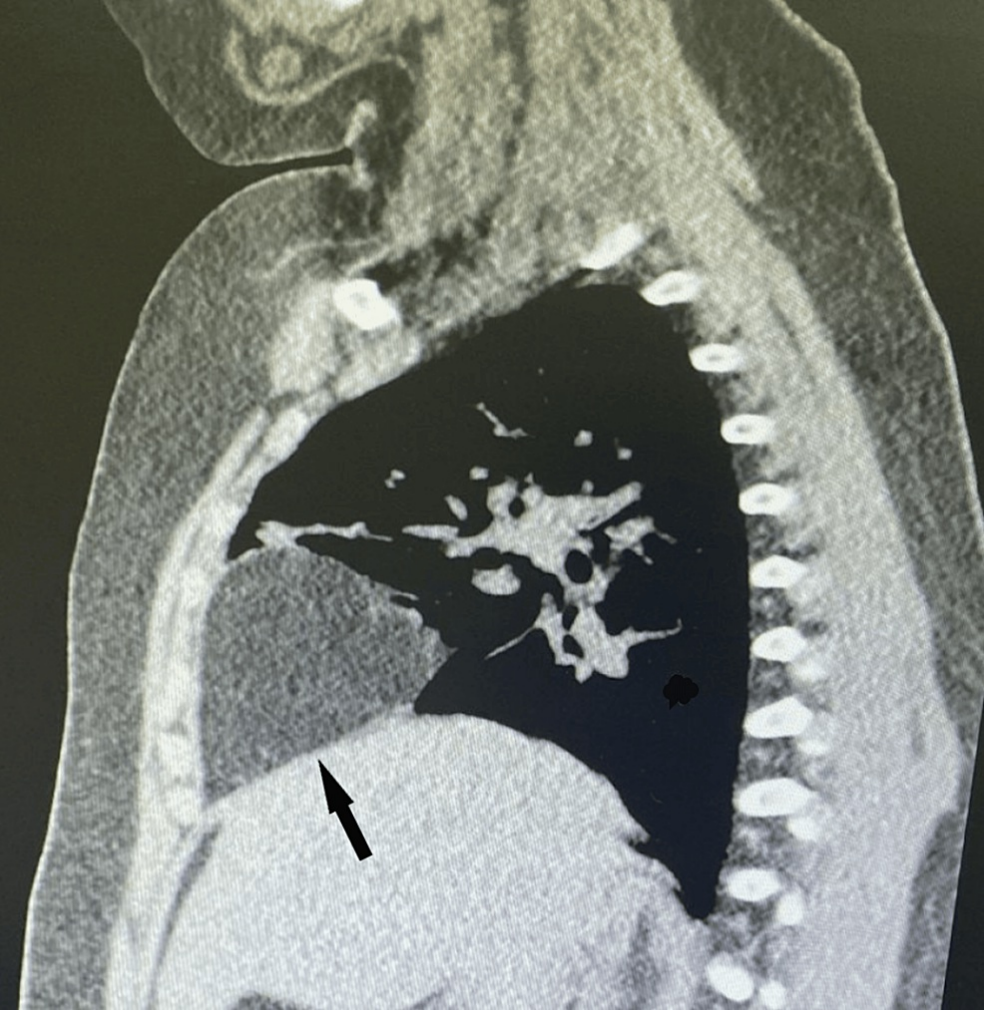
Computed tomography with contrast showing herniation of omental fat in the anterior mediastinum (arrow).
